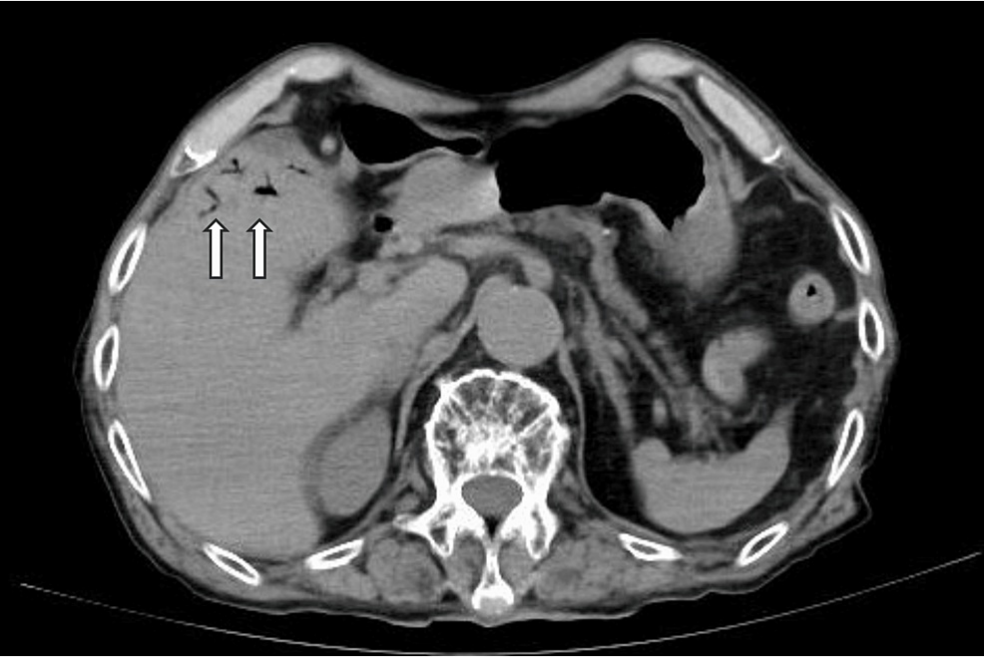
Portal emphysema in the portal vein shown on abdominal CT
